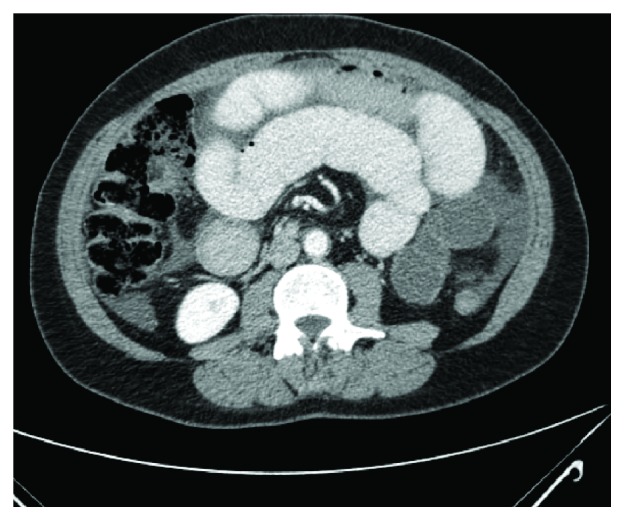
CT of the abdomen. Distended loops of small intestine in the right and left upper and middle quadrants, consistent with small-bowel obstruction. There is an associated moderate volume of interloop ascites within the small-bowel mesentery.
